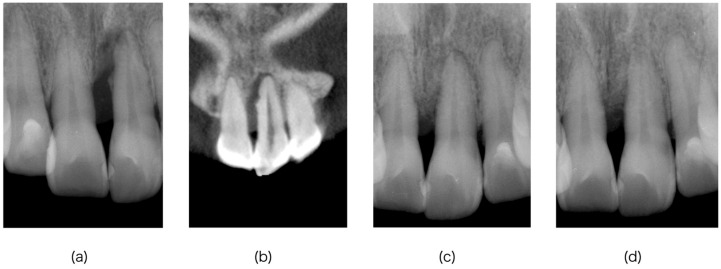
Representative clinical case demonstrating application of the proposed framework. (a) Initial periapical radiograph showing marginal bone loss extending toward the apical region. Clinical examination revealed a localized deep periodontal pocket with calculus accumulation, while the crown was intact without caries or defective restoration. The tooth responded normally to cold testing and electric pulp testing (EPT), with no spontaneous pain or percussion tenderness. (b) Cone-beam computed tomography imaging demonstrating that the radiolucency, although extending close to the apex, was not centered on the root apex and showed no features consistent with endodontic pathology. No sinus tract or coronal leakage was detected, and the lesion was interpreted as periodontal in origin (V_1_/IC^−^). (c) Three-month reevaluation after periodontal therapy showing clinical improvement without signs suggestive of pulpal pathology. (d) One-year follow-up radiograph demonstrating periodontal healing without development of periapical pathology. The tooth remained responsive to cold testing and EPT, confirming maintained pulp vitality.
